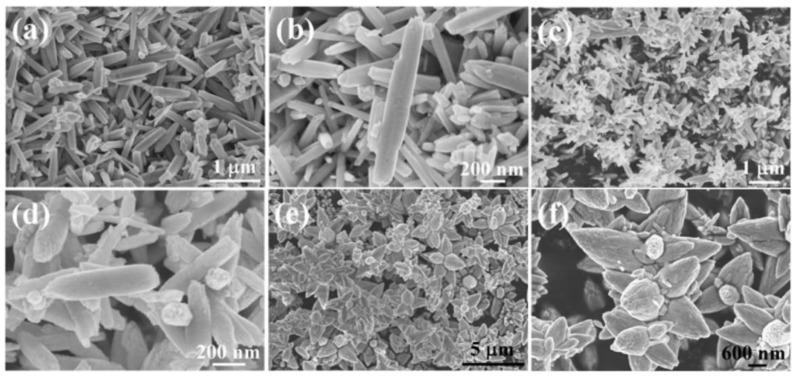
SEM images of ZnO products synthesised from zinc acetylacetonate hydrate with the Zn/HEPES molar ratio of 1:5 prepared under different pH values: 7.4 (a,b); 8.4 (c,d); 9.4 (e,f). Products synthesised by the microwave and ultrasonic wave combined method. Reprinted from [844], Copyright (2013), with permission from Elsevier [OR APPLICABLE SOCIETY COPYRIGHT OWNER]. All rights reserved. In order to re-use permission must be obtained from the rightsholder.
